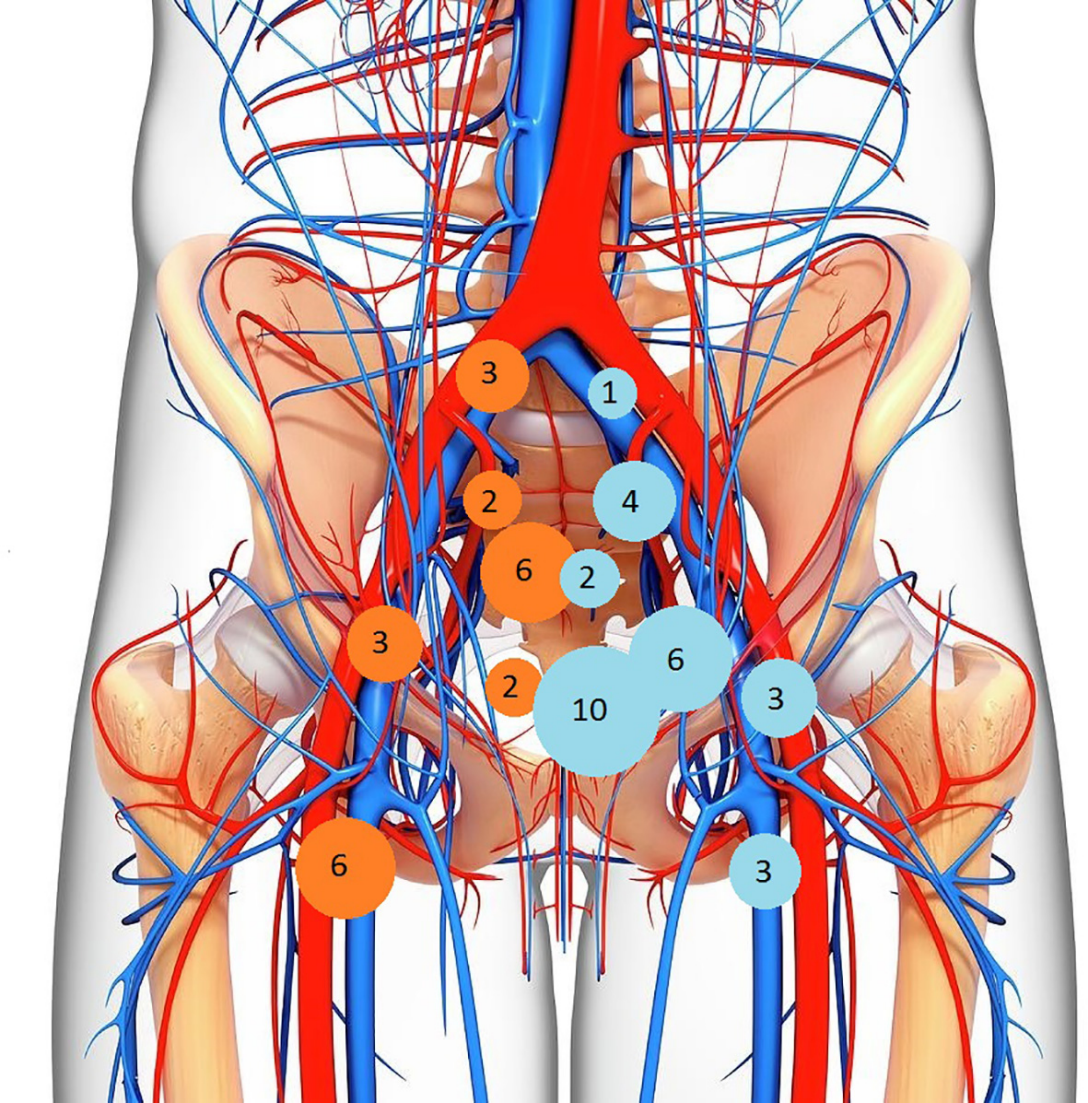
Mapping of cases with PET-positive (orange) and MRI-positive (light-blue) lymph nodes.
